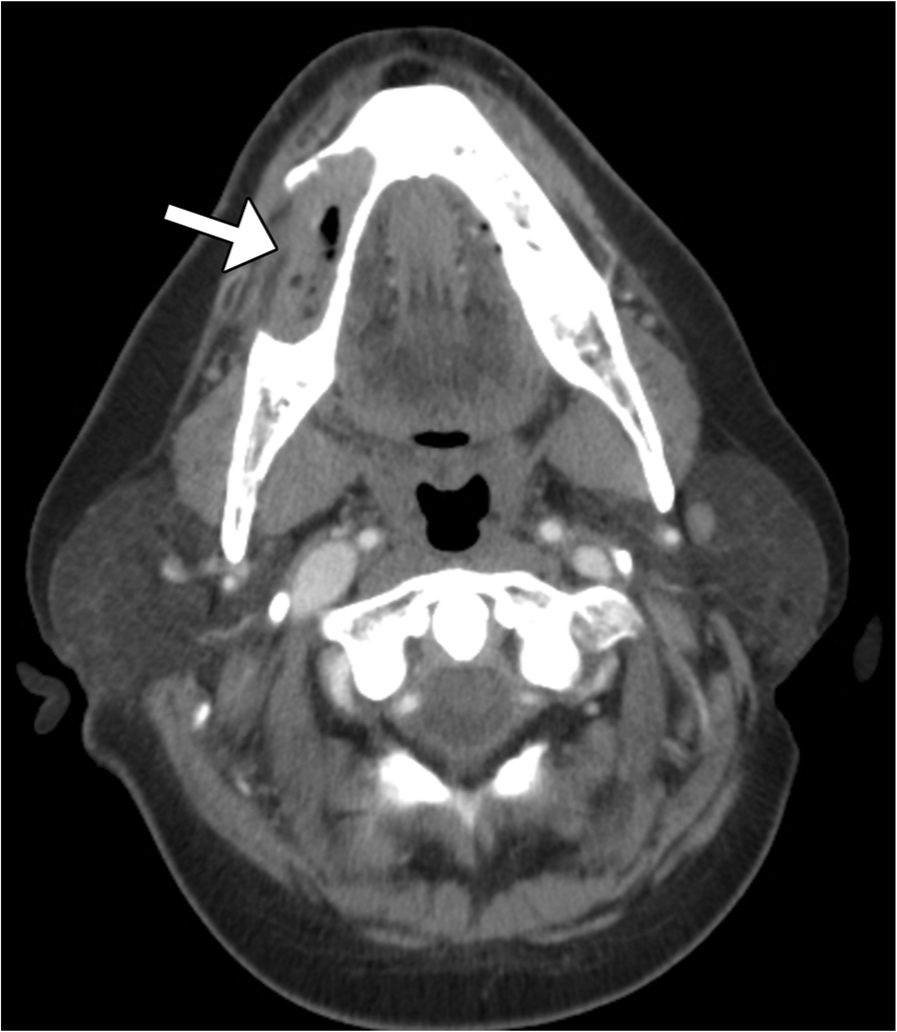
Ameloblastic carcinoma. Axial CT image shows a lytic lesion involving the right mandible (arrow)
